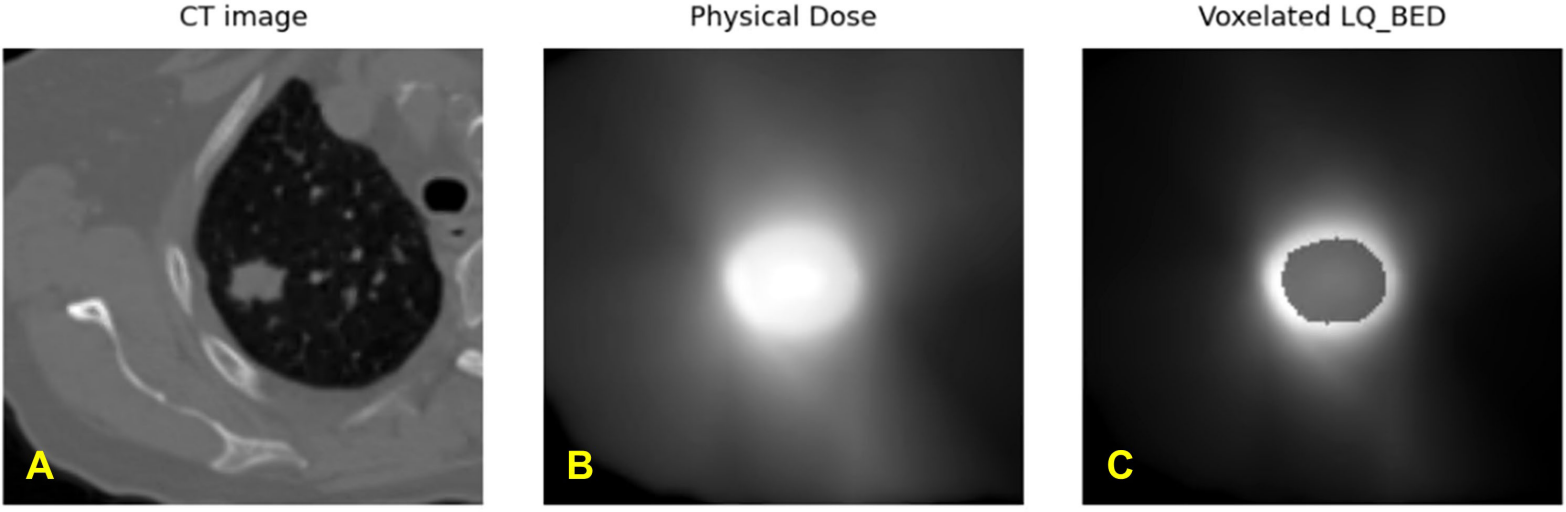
Exemplary representation of (A) planning CT image, (B) physical dose distribution extracted directly from the treatment plan, and (C) calculated biologically effective dose (BED) distribution from one patient.
